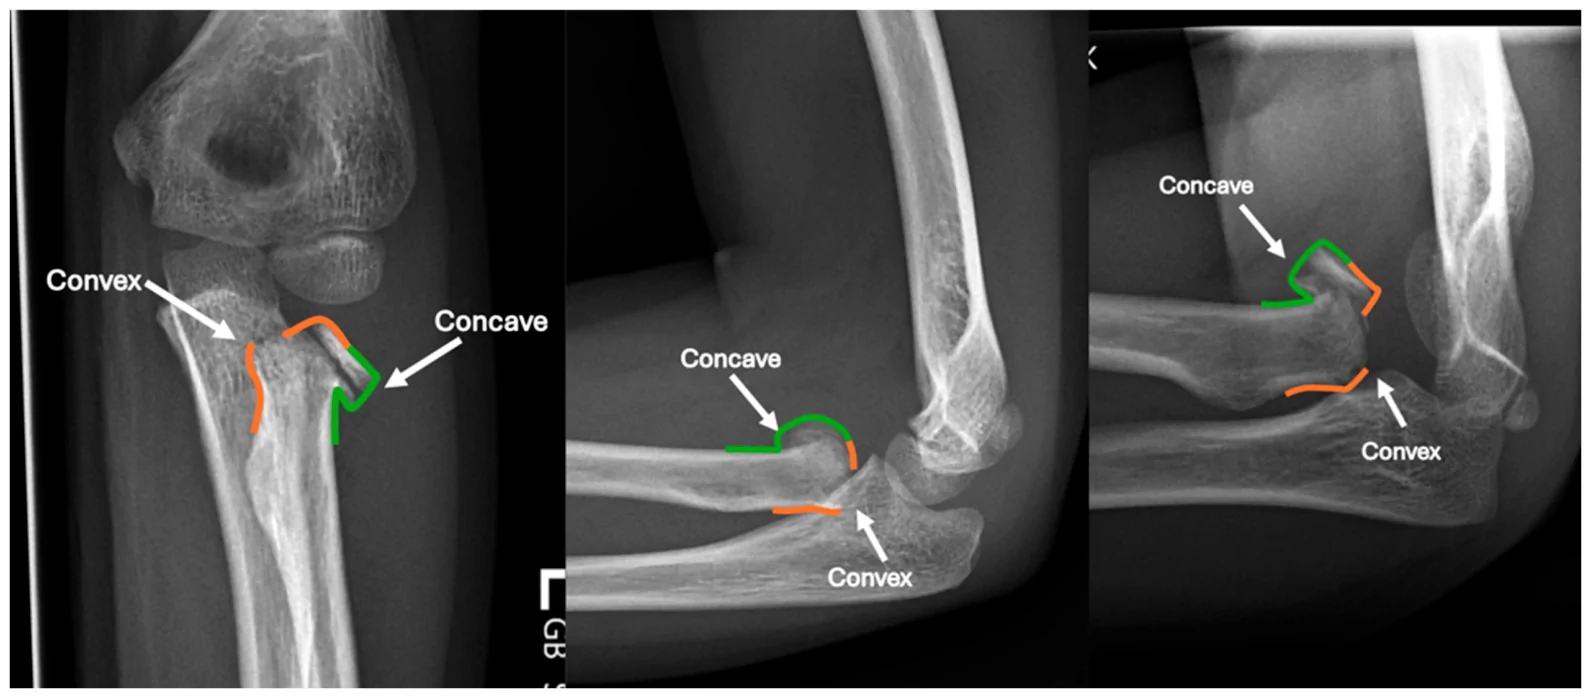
Annotated radiographs demonstrating the concave and convex sides of the radial neck fracture, with the intact periosteum expected on the concave fracture side (green) and the torn periosteum expected on the convex side (orange).
